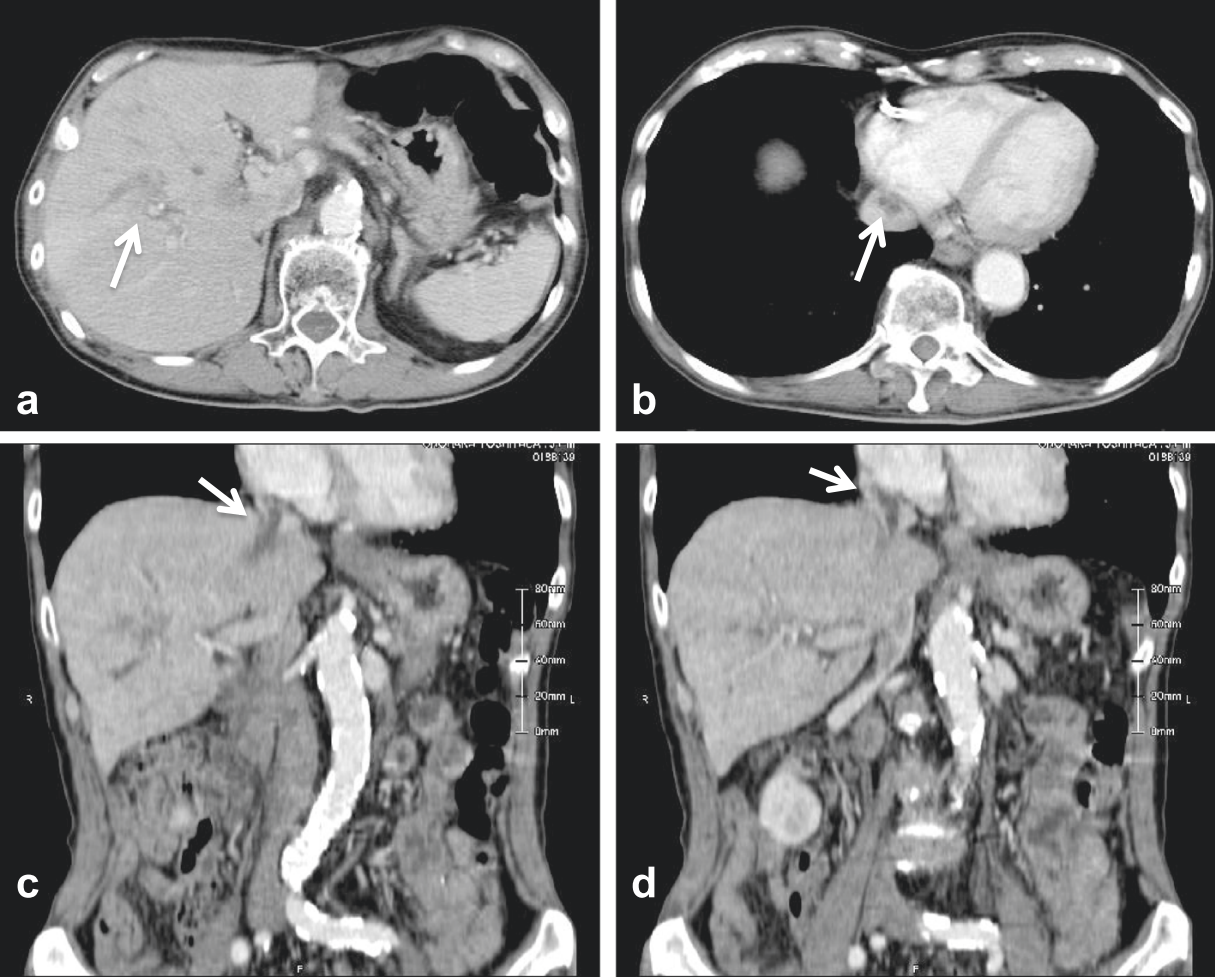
Follow-up computed tomography on hospital day 25. a The abscess was reduced in size (*arrow*) b, c, d The thrombus enlarged further and extended close to the right atrium
